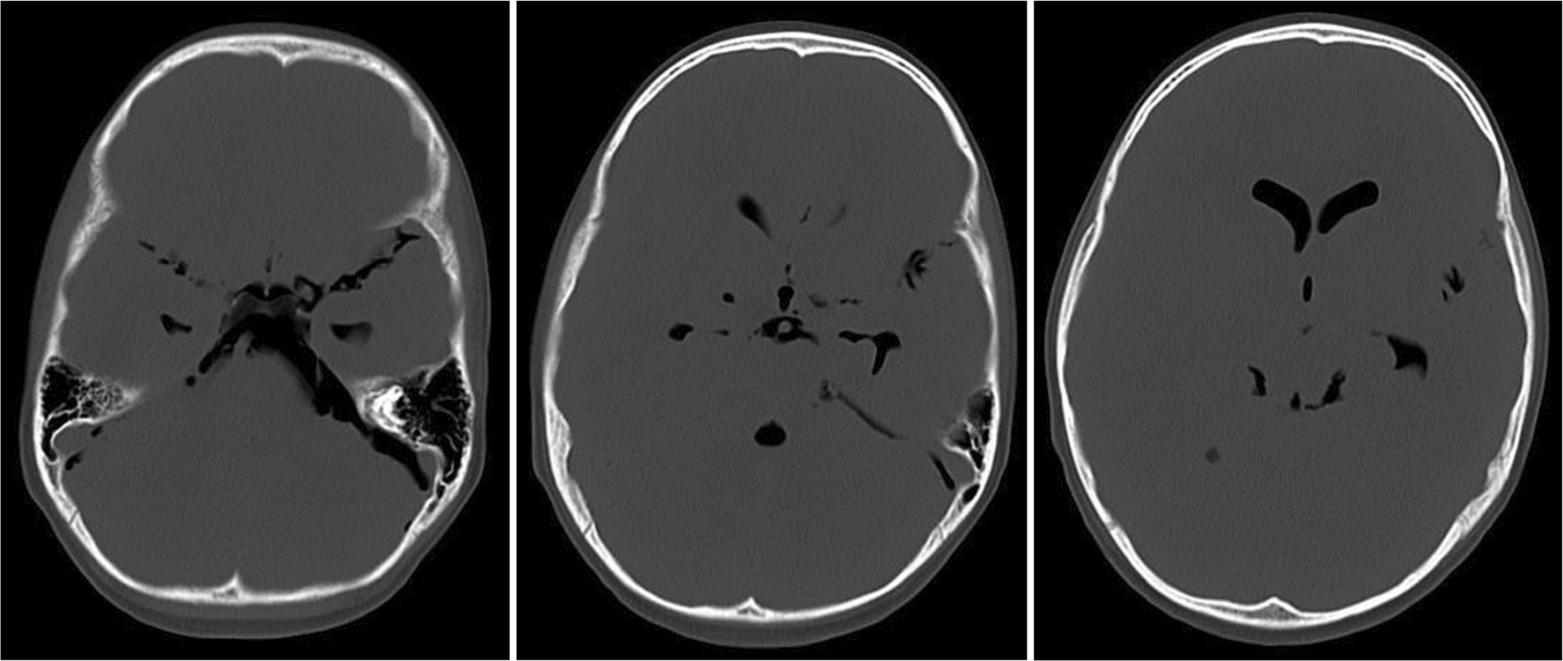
Cerebral CT, axial section, bone setting
